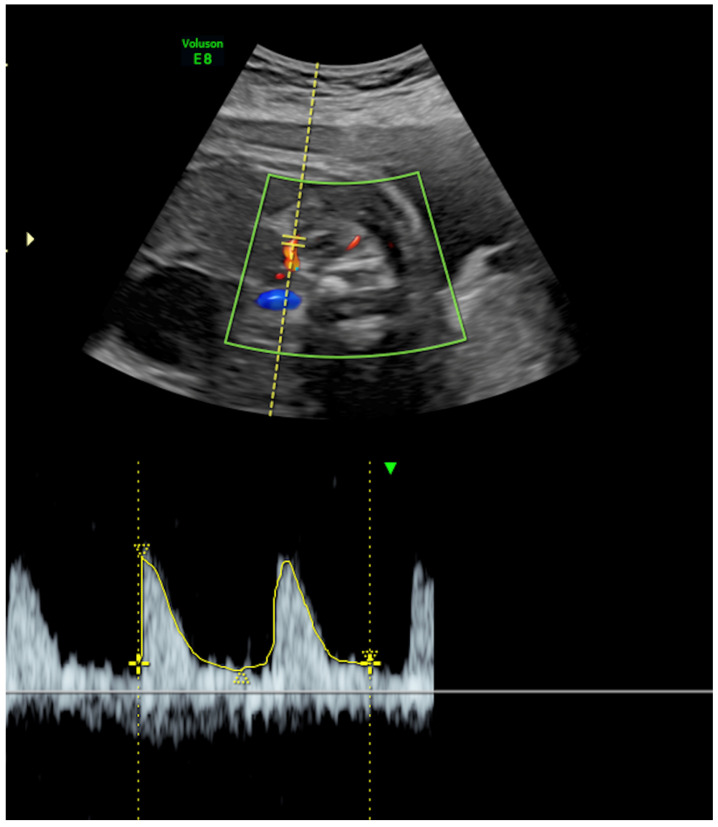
Middle adrenal artery Doppler velocimetry.
